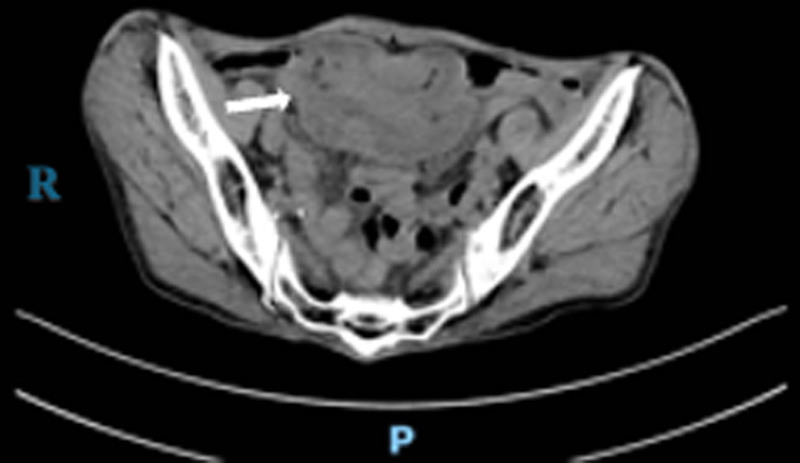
Abdominal computed tomography scan showing ileocecal intussusception (white arrow).
